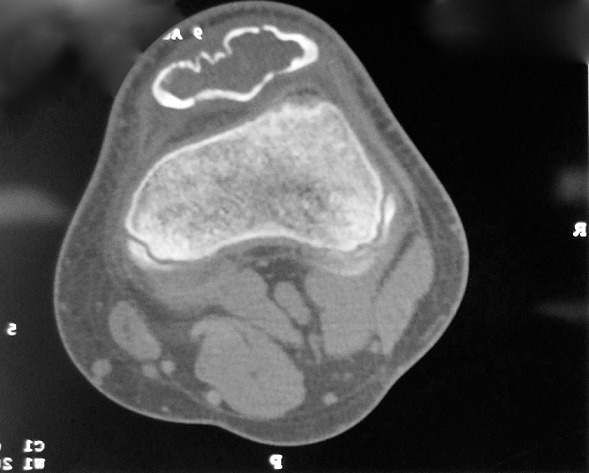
Sagittal CT scan image showing a multi-loculated lesion in the patella
